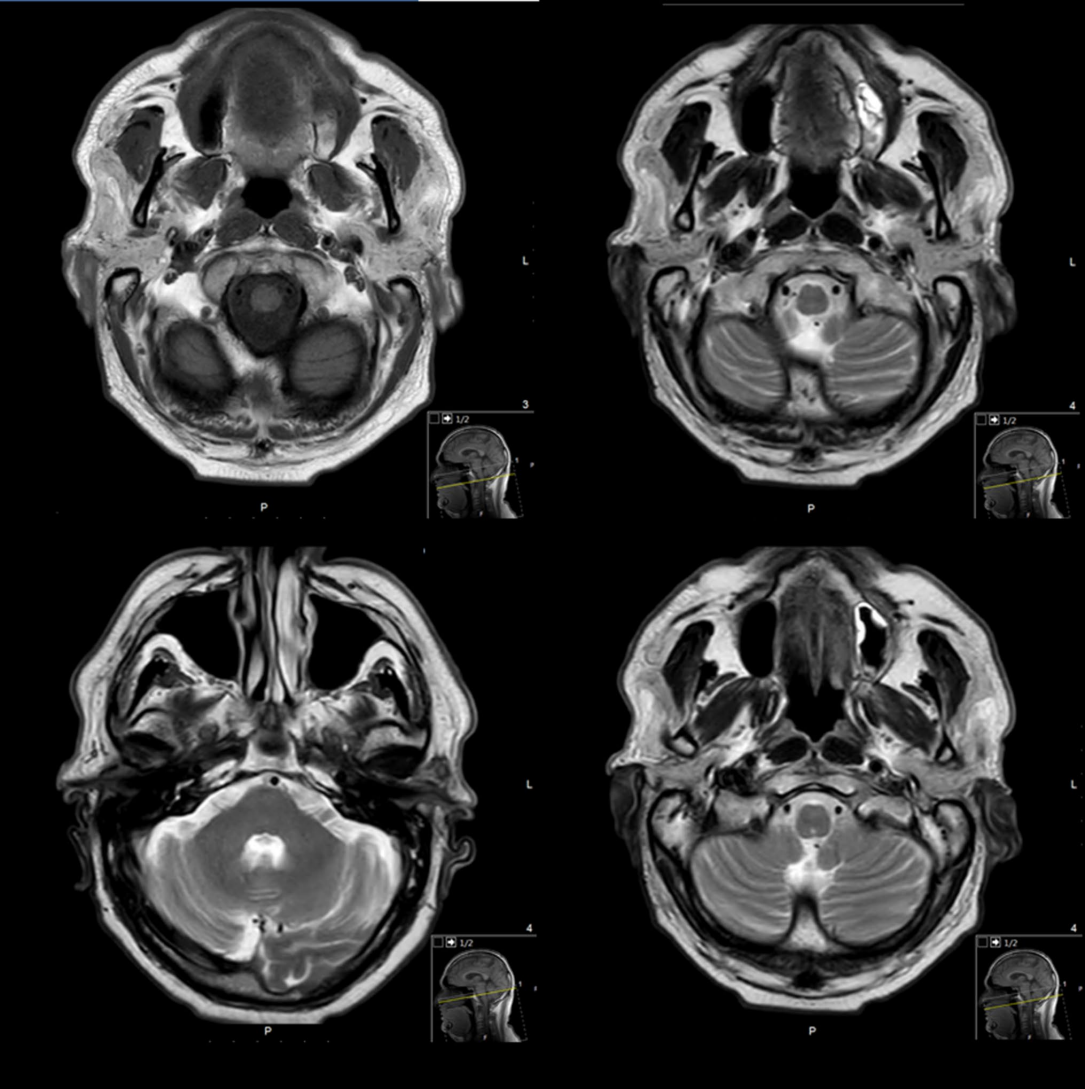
MRI T1 and T2—after 2 cycles of the neoadjuvant treatment (after 8 weeks) shows significant decrease in the tumor or even its disappearance, attained a complete remission
